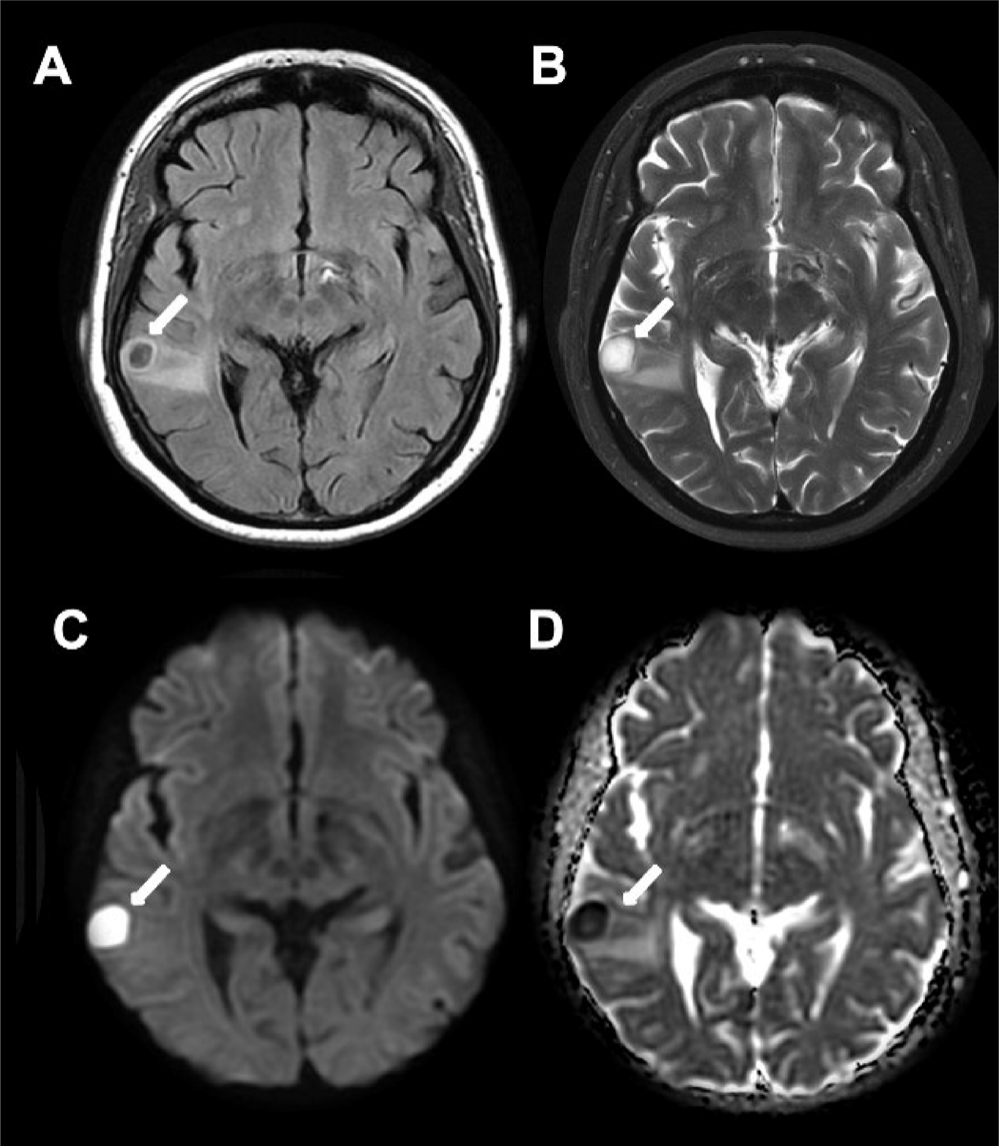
Brain MRI in axial FLAIR (A), T2-weighted (B), B1000 diffusion (C), and ADC map (D). A pyogenic abscess surrounded by vasogenic edema (arrows) in the right superior temporal gyrus is detected.
